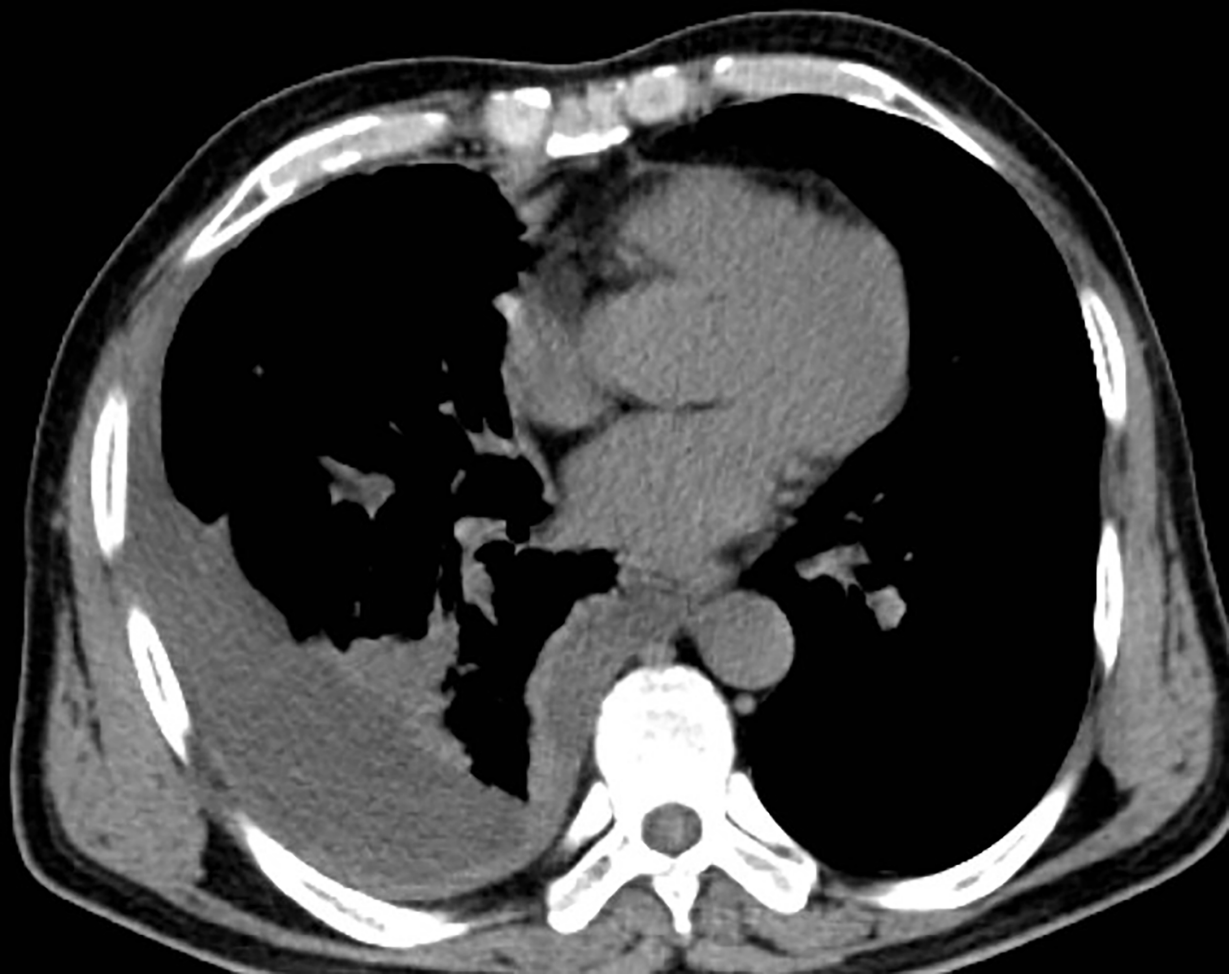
CT before immunotherapy.
